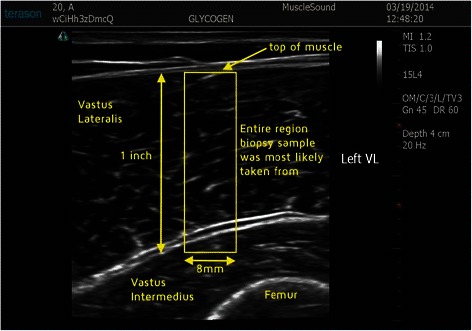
Ultrasonic scan from a subject with the rectangle area representing where images were segmented to isolate the muscle area under analysis using a center crop within the muscle section 25 mm from the top of the muscle sheath.
